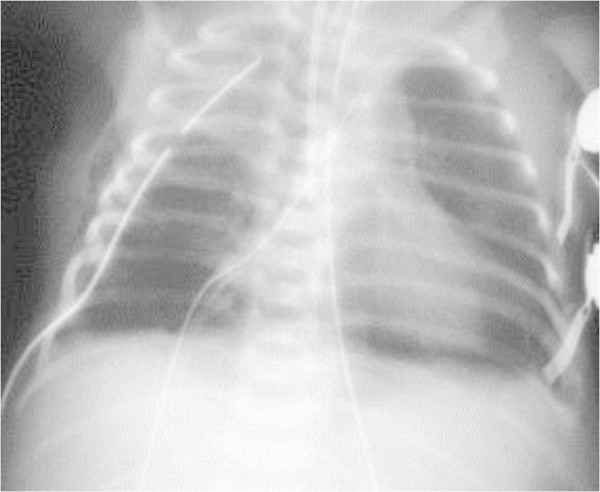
Postoperative frontal radiograph after repair of the peritoneopericardial hernia. Resolving atelectasis of the upper lobe of the right lung is apparent. The mediastinum and both leaves of the diaphragm are normal. The endotracheal tube, nasogastric tube, right chest tube and pericardial tubes are visible.
